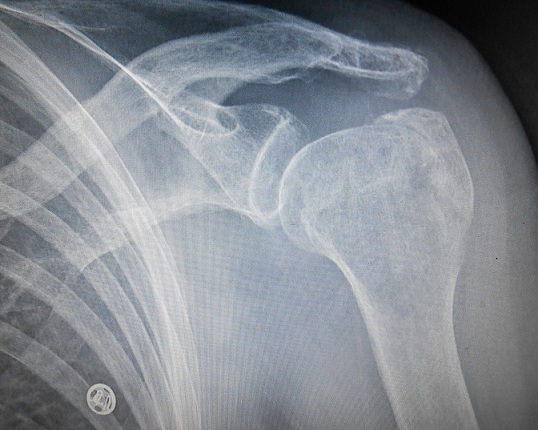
La radiographie de face à 3 mois de la réduction
